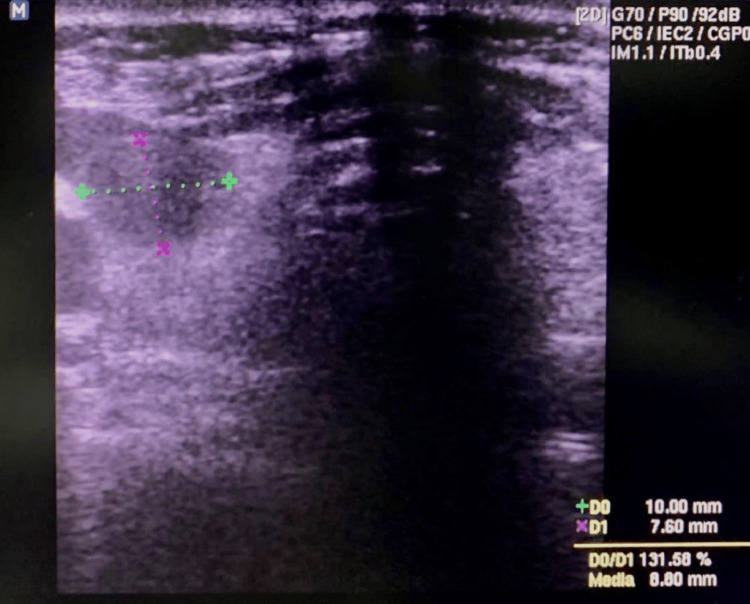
Neck ultrasound after aspiration of fluid from the cystic space
